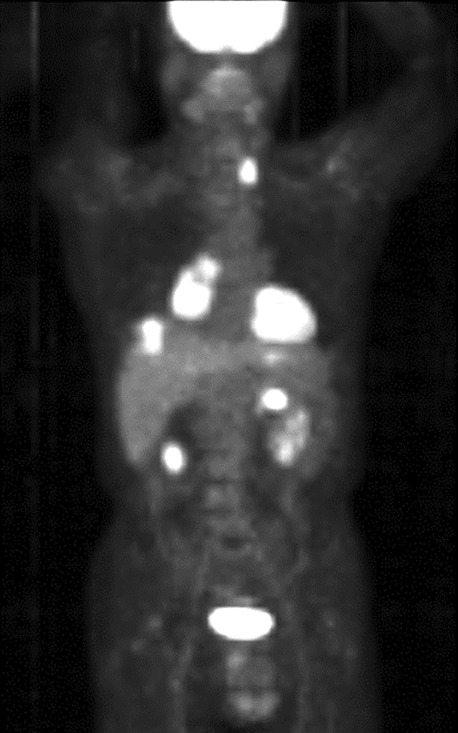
Fluorodeoxyglucose (FDG)‐positron emission tomography revealed high FDG uptake in the left thyroid lobe and the ascending colon as extrapulmonary findings.
